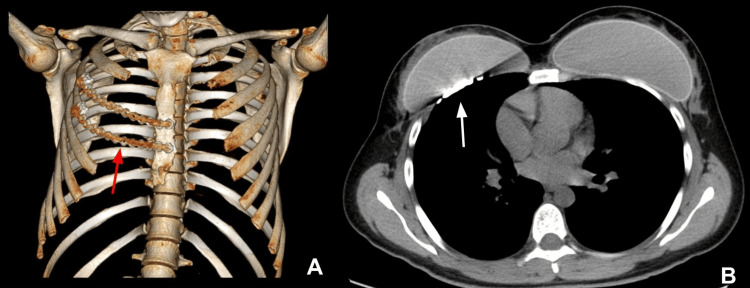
(A) Three-dimensional reconstruction of the rib cage demonstrates a rib prosthesis (red arrow) in the right hemithorax. (B) Postoperative control computed tomography following resection of the mass in the right rib cage shows complete removal of the previously described lesion with no evidence of suspicious residual or recurrent lesions at the surgical site. Osteosynthesis material is visualized within the rib cage (white arrow).
